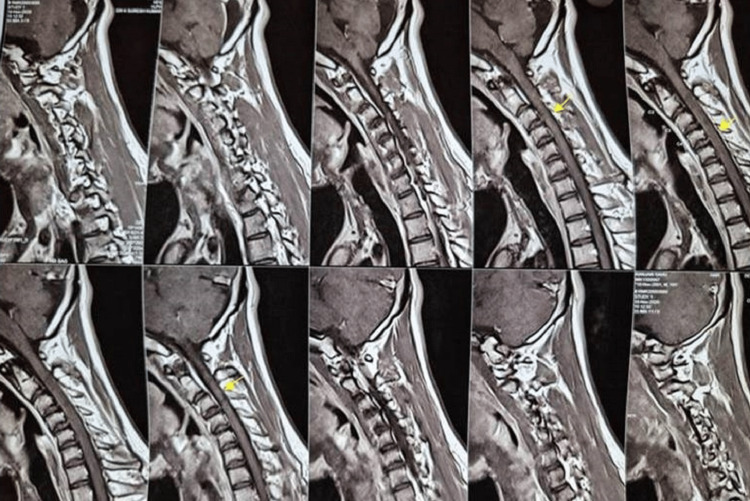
Normal appearing MRI sequence of the cervical region with neck flexion.
